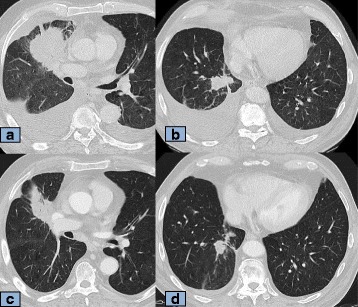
Thoracic computed tomography scan comparison after the first and the fourth nivolumab infusion. Computed tomography scan, axial views through the middle and lower lung lobes before the first nivolumab injection (a and b) and after four courses of nivolumab and oral corticosteroid therapy (c and d). Both the primary tumor in the middle lobe and the metastasis in the lower lobe of the right lung shrank substantially (from 80 to 60 mm and from 23 to 16 mm, respectively). The amount of fluid in the two pleural cavities also decreased
